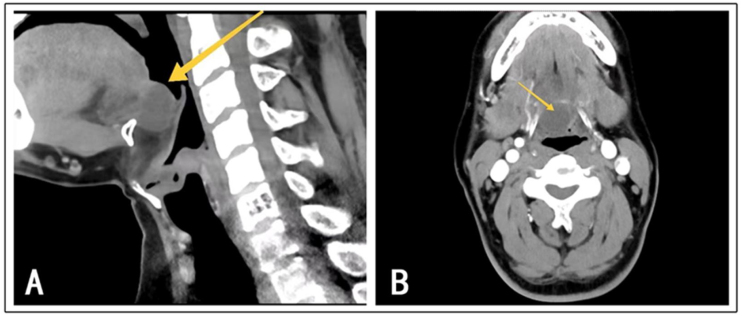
(A) Case 1: Location of the intraoral thyroglossal duct cyst as shown on the CT sagittal plane. (B) Case 1: Location of the intraoral thyroglossal duct cyst as shown on the CT axial plane.
